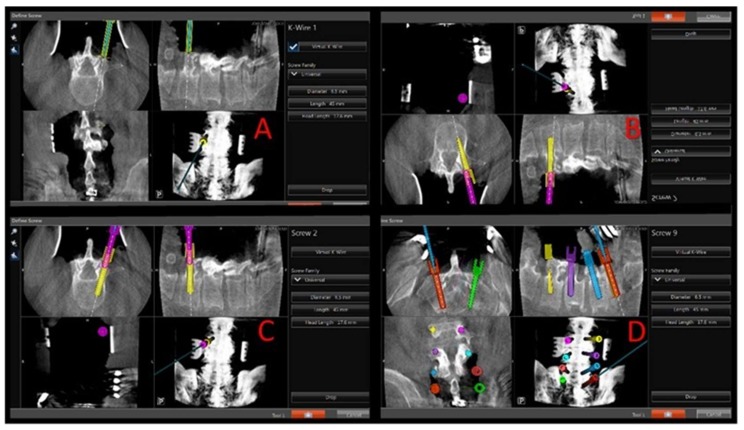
Intraoperative views of navigation screen showing steps of virtual screw placement. (A) Navigated Stryker drill with 3 mm match stick drill bit at anatomical entry point with screen projection showing a 6.5 × 50 mm virtual screw. (B) Navigated Stryker Driver with 5.5 tap accessing the vertebral body through the pedicle with screen projection again showing 6.5 × 50 screw. (C) Navigated Stryker driver with 6.5 × 50 screw with screen projection showing the same 6.5 × 50 virtual screw. The instruments used at each step are color-coded to avoid confusion on the navigation monitor while advancing into the pedicle and vertebral body (match stick drill bit in “A”, green. Tap in “B”, yellow. Screw in “C”, pink. (D) Screen shot showing final screw placement before intra-operative CT done to confirm placement.
